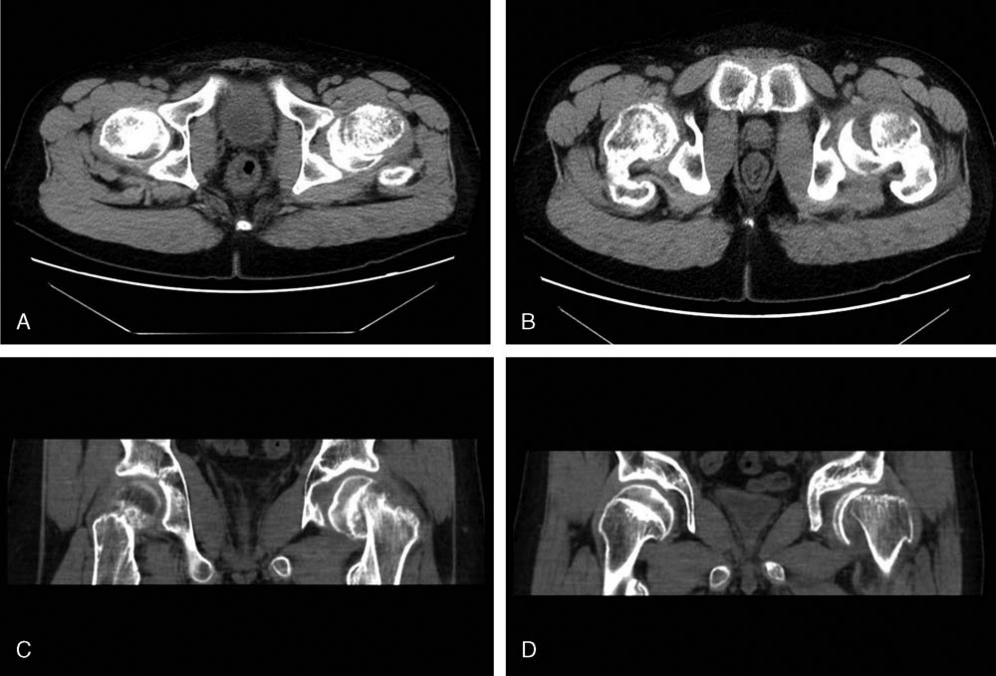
a-d. Computed tomography of both hips performed after the pain had worsened. The epiphysis has slipped backward on the left side as can be clearly observed in the coronal (A, B) and axial (C, D) scans.
